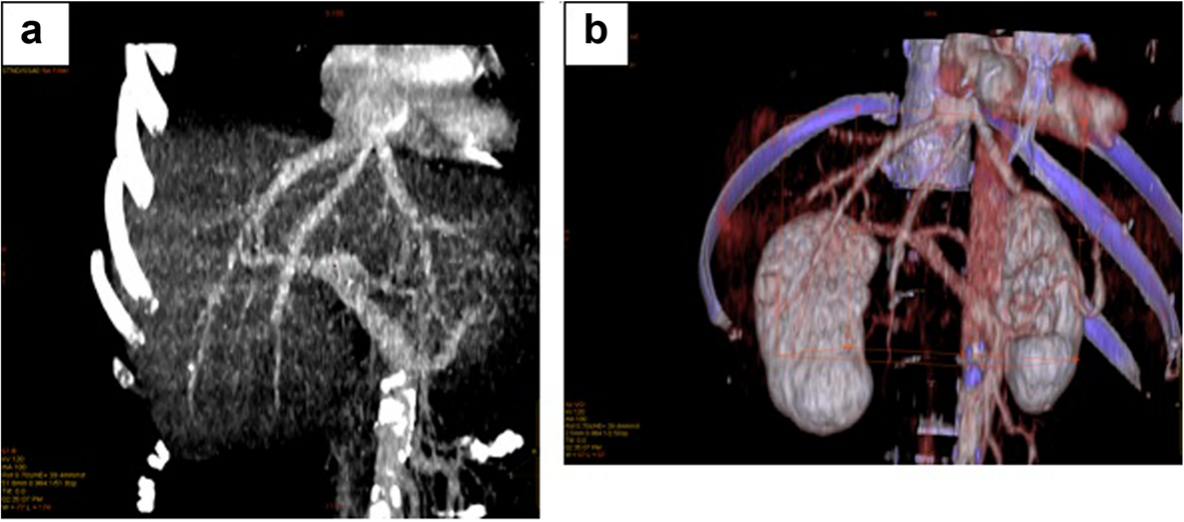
Post-transplant computed tomography scans: a Anterior CT image of hepato-atrial (atrial-caval) anastomosis; b 3-Dimensional computed tomography reconstruction of the vascular image presenting the hepato-atrial anastomosis
